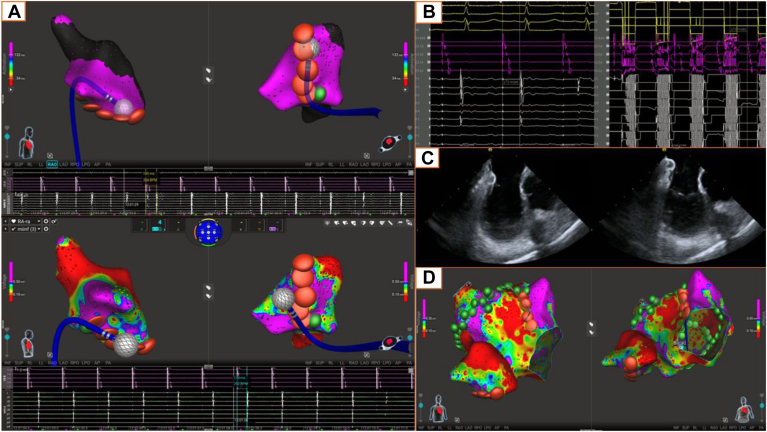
A: Top: Sphere-9 catheter positioned directly on the CTI ablation line with observed local split potentials (185 ms) postablation. Bottom: Final CTI ablation map with RF lesions (*red*) and PFA lesions (*green*) superimposed on the baseline right atrial voltage map. PFA was performed only at the posterior CTI near the inferior cavoatrial junction, far from the right coronary artery. B: Differential atrial pacing using a proximal coronary sinus catheter and Sphere-9 catheter positioned just lateral to the CTI ablation line. The postablation isthmus conduction time is 170 ms in both directions, consistent with bidirectional CTI block (the preablation isthmus conduction time was 66 ms). C: Intracardiac echocardiography images acquired during CTI ablation, with the Sphere-9 catheter located on the CTI. D: Baseline right and left atrial voltage maps with the corresponding RFA and PFA lesions. In total, 85 PFA lesions and 10 RF lesions were applied to complete redo pulmonary vein isolation and posterior wall isolation (PFA), create an anterior mitral line with RFA + PFA, a CTI line (RFA + PFA), and superior vena cava isolation (PFA; not shown). CTI = cavotricuspid isthmus; PFA = pulsed-field ablation; RFA = radiofrequency ablation.
